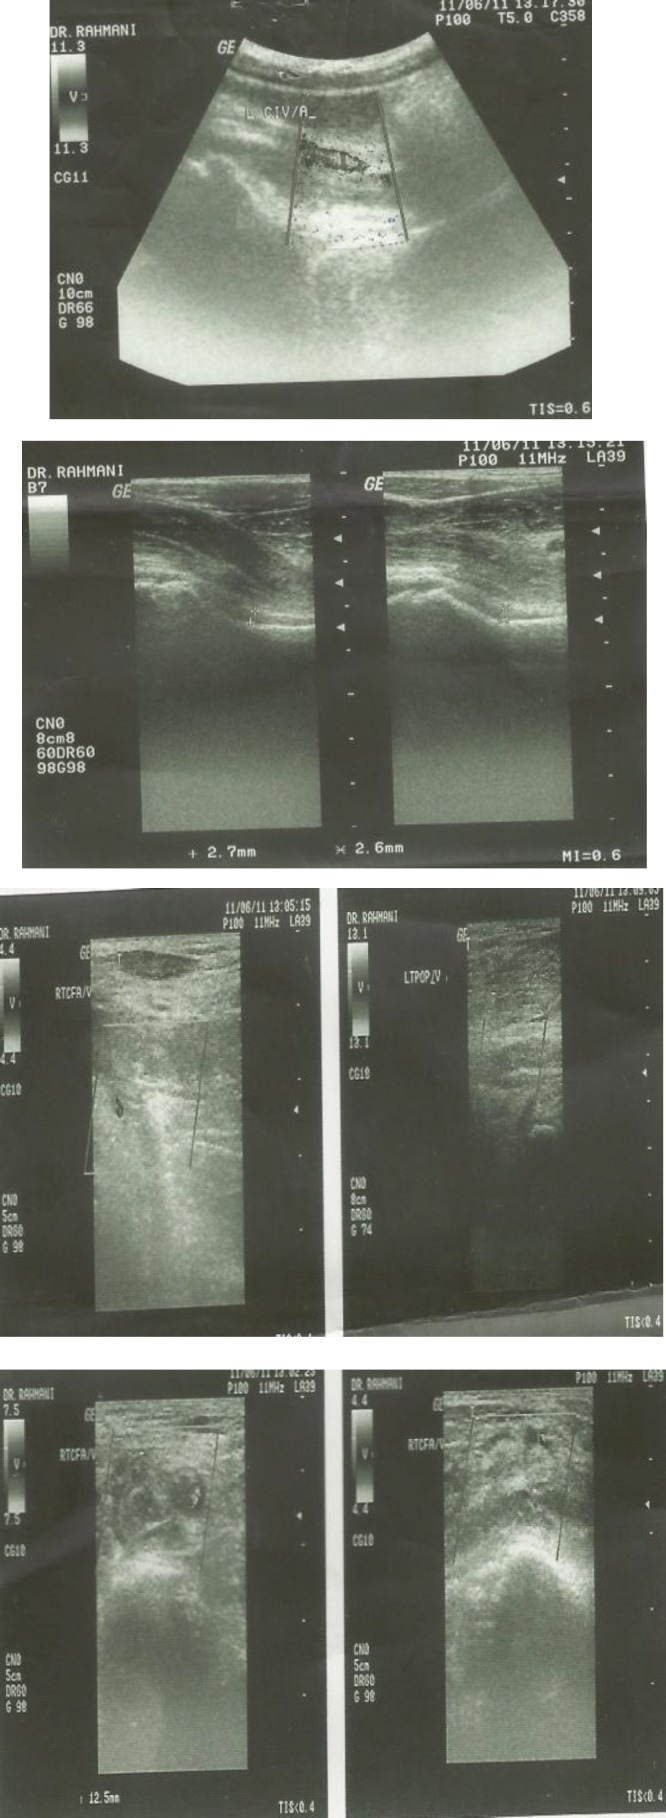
Deep vein Doppler ultrasound of left lower extremity showed that common iliac vein common femoral vein, external iliac vein and superficial and deep femoral vein & popliteal vein were enlarged and contained with echogenous clot
